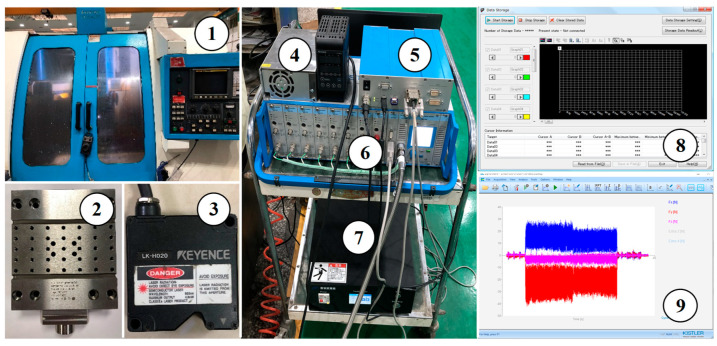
Cutting force experimental equipment and measurement software. (1) VMC850 machine tool, (2) Kistler 9119A multicomponent dynamometer, (3) Keyence LK-H020 sensor head, (4) Keyence LK-G5000 laser displacement sensor, (5) Kistler 5697A data acquisition system, (6) Kistler 5080A charge amplifier, (7) ultrasonic generator, (8) LK-Navigator2 (version 01.04.0.3), and (9) Dynoware software.
